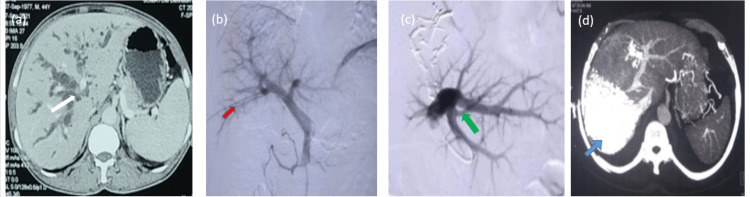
Sequential images of the process of portal vein embolization (PVE). (a) CT scan of the abdomen showing mass at the hepatic hilum (white arrow) causing complete obstruction of the bile duct with dilated intrahepatic biliary radicles. (b) Percutaneous portogram via the right posterior portal vein (red arrow) showing all branches of the portal vein. (c) Selective embolization of the right portal vein and the left medial portal vein with visualization of only the left lateral portal vein (green arrow). (d) Post-procedure CT scan of the abdomen after three weeks of PVE showing hypertrophy of left lobe with atrophic right lobe filled with lipiodol particle (blue arrow) used during PVE.
